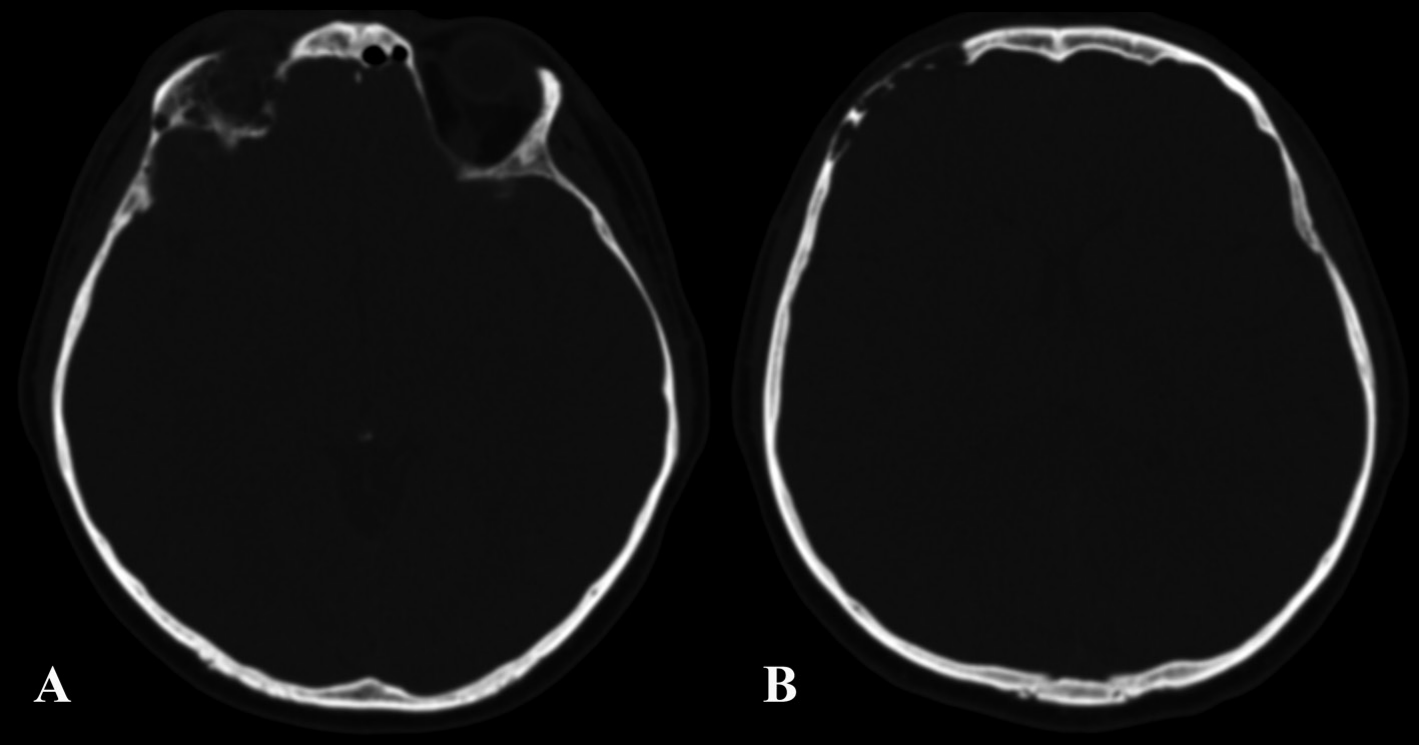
Pre-operative CT showing the involvement of the right orbital roof (A) and the right frontal bone (B) by the lesion.
